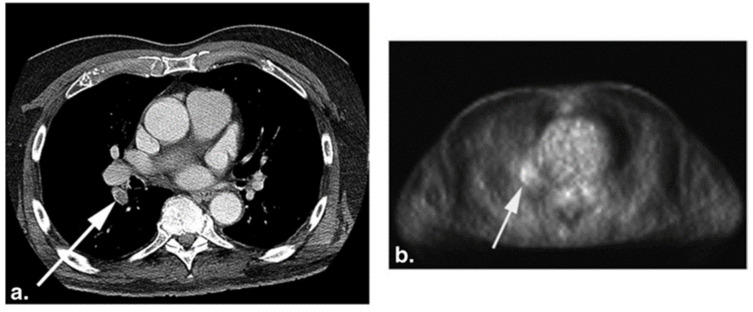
CT (a) and the corresponding FDG-PET (b) images of a 75-year-old woman with a history of melanoma. The white arrows point to pulmonary embolism (PE) present in the right lower lobe segmental artery. Increased FDG uptake (b) is seen at the location of PE on the PET image (from Flavell et al. [119] with permission).
